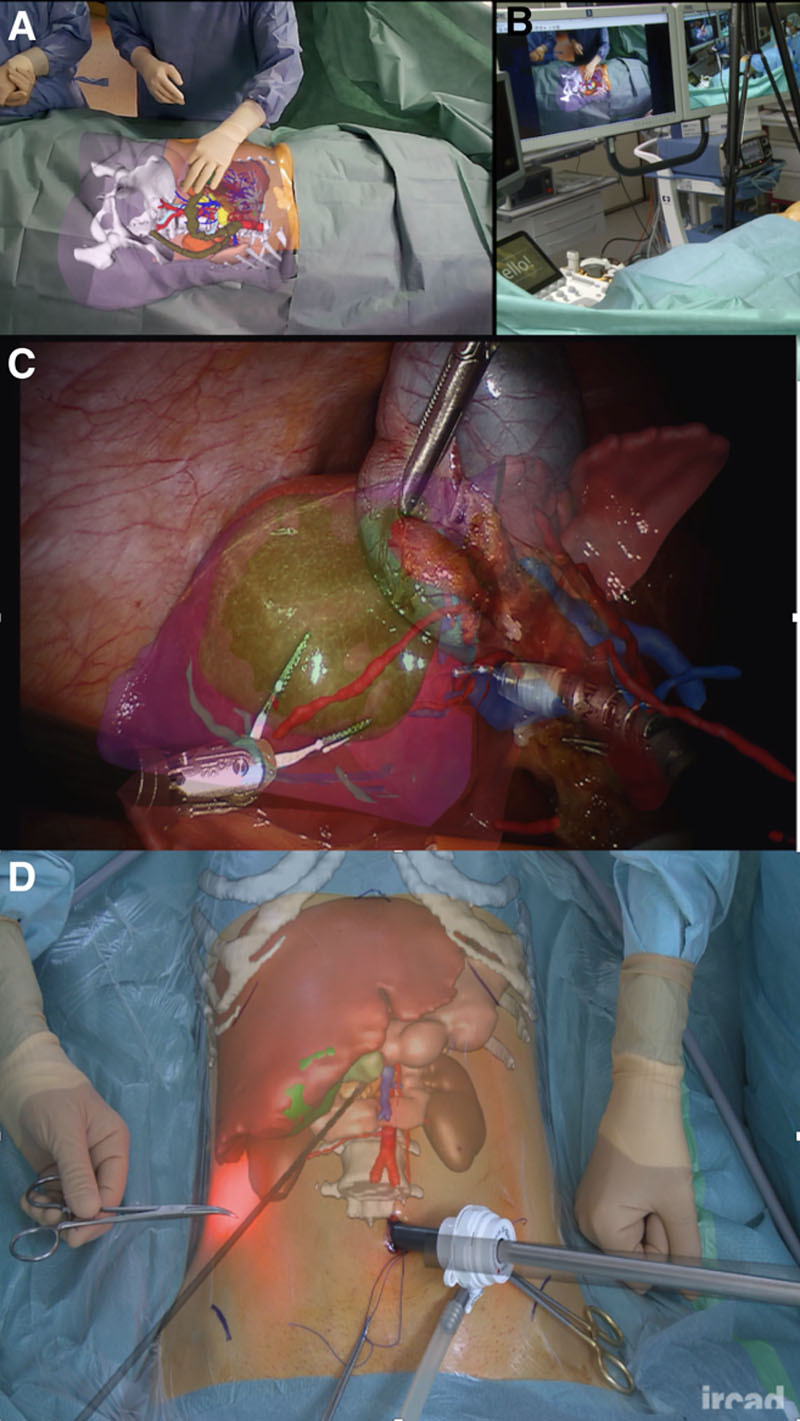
Image-guided surgery and intervention. During the planning phase, a contrast-enhanced computed tomography scan acquisition is post-processed and segmented to obtain the 3-dimensional models employed to provide augmented reality. A and B, Show the use of the visual augmentation assisting the initial phase of a laparoscopic approach. C, Shows the same augmentation overlaid in the display used for laparoscopic vision, and (D) the possible switching from one to the other augmentations throughout the procedure.
